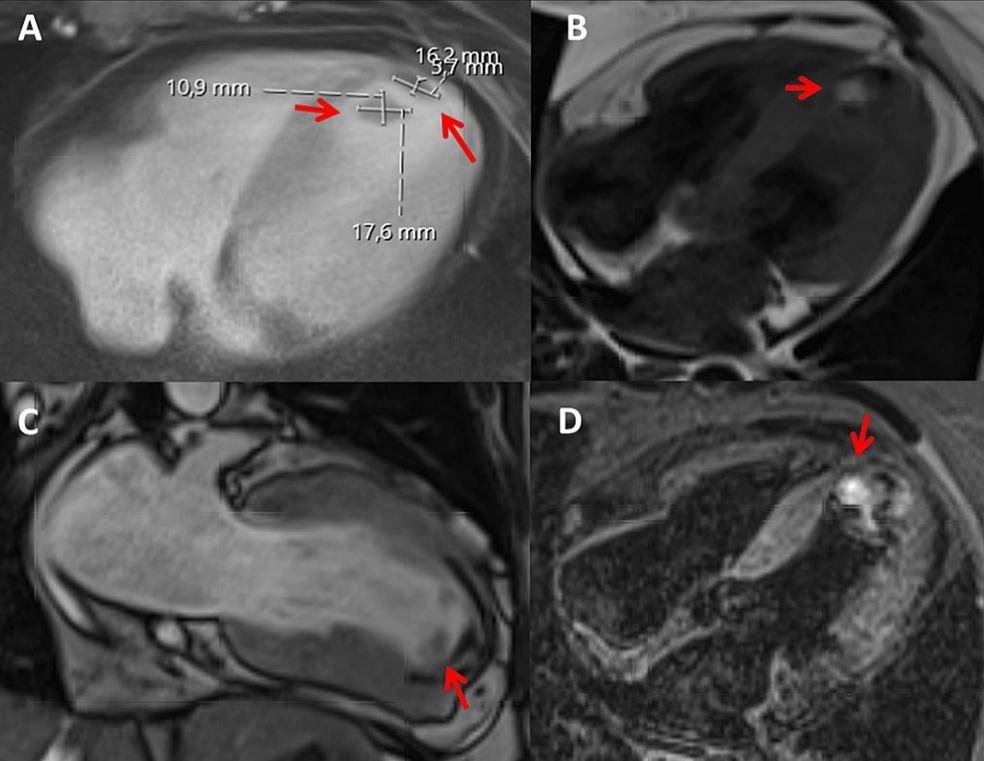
Cardiac MRI of the index patient’s heart at age 54 showing myocardial thickening and two organized apical thrombi at different modalities and projections (A-D) (red arrows). MRI: magnetic resonance imaging
